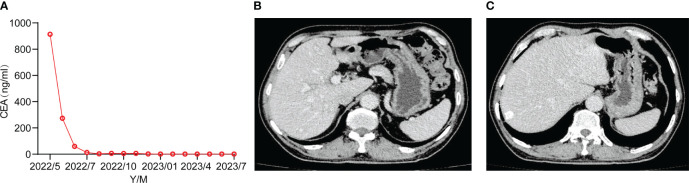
(A) Tumor markers returned to normal. (B, C) Follow-up CT in July 2023 assessed the stability.
